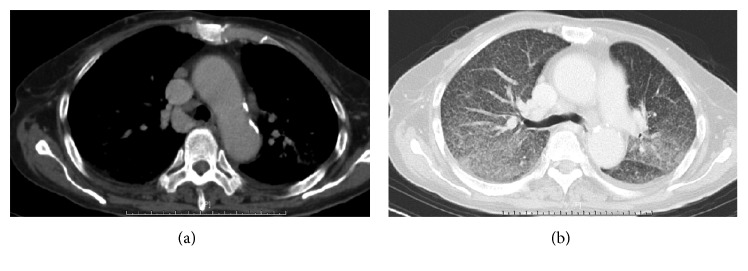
(a) Computed tomography (CT) of chest in soft tissue window revealed lymphadenopathy over mediastinum and (b) multiple tiny nodules along with alveolar infiltration in lung window over bilateral upper lung fields, diagnosed as military tuberculosis combined with pulmonary tuberculosis.
